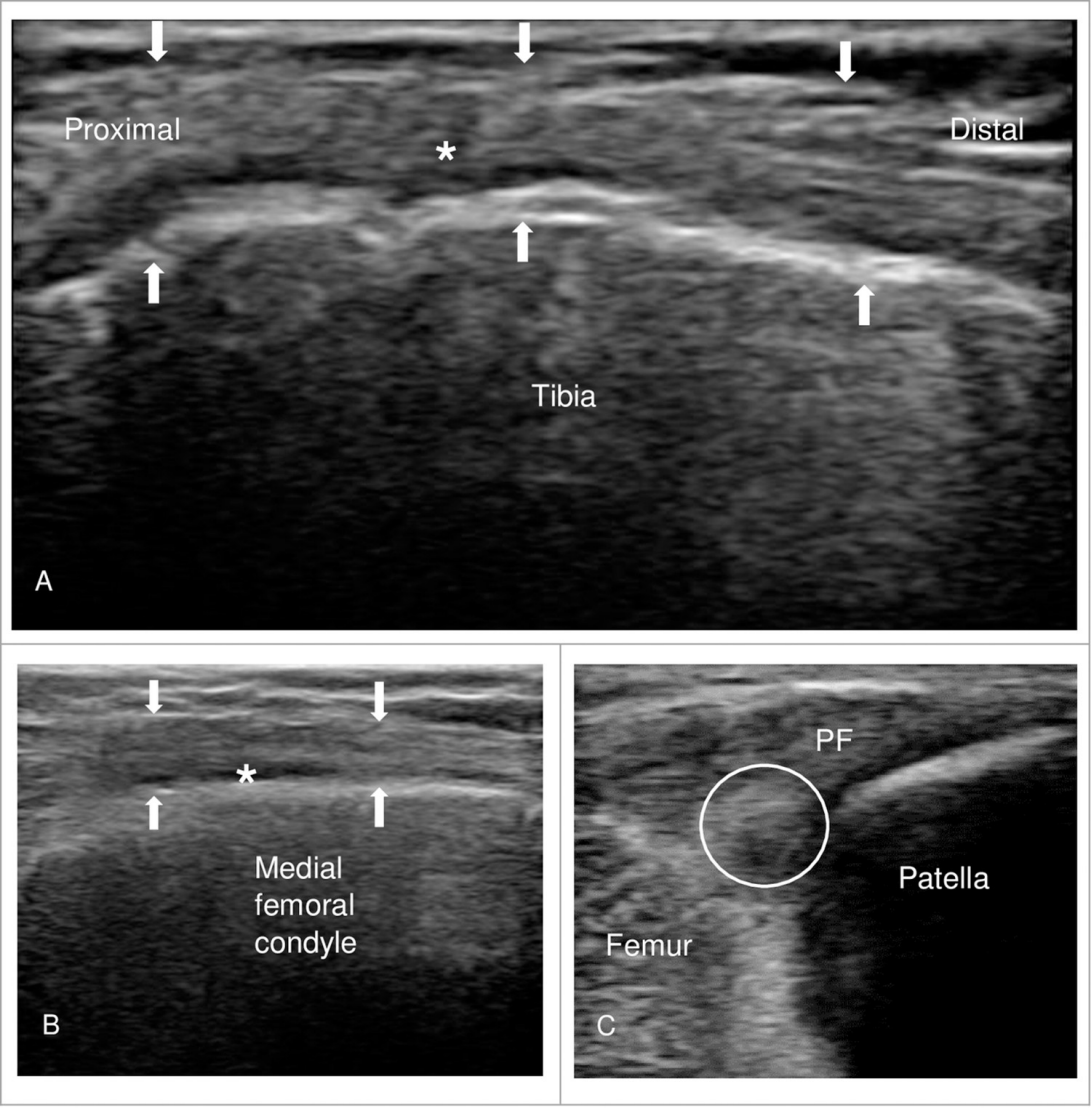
Sonographic examination images of a patient with KOA. (A) Coronal plane of the medial collateral ligament (MCL: white arrow) displaying mucoid degeneration (white star*); (B) Hypoechoic appearance of the medial collateral ligament (white arrow) in transverse plan displaying effusion (white star*); (C) Longitudinal view of the patellofemoral ligament (PFL) displaying hyperechoic change in the region of patella (white circle).
